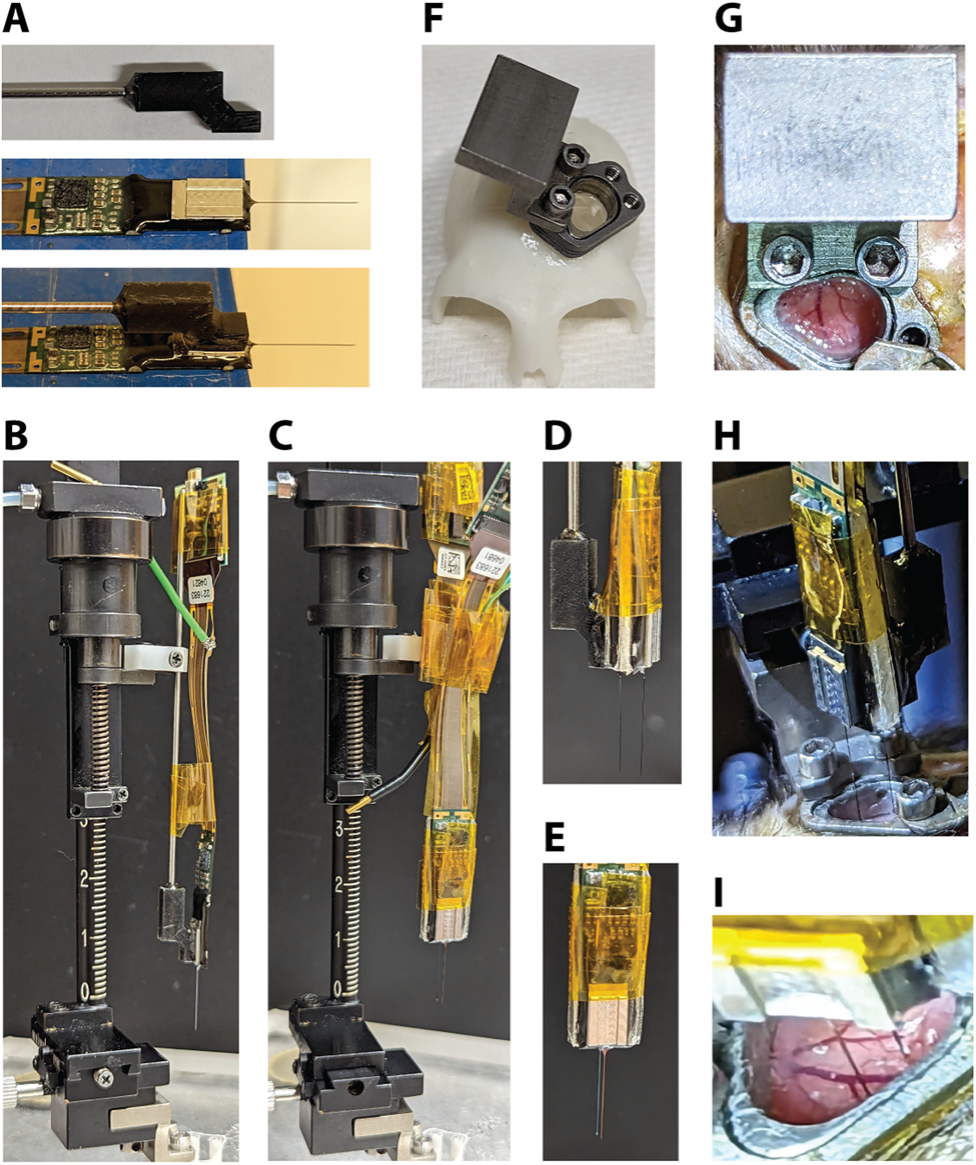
Neuropixels recording setup. *A*, A custom 3D-printed single probe holder with a metal rod is glued to the base of the Neuropixels probe. Ground and reference wires are also attached. *B*, The rod is held by the clamp of the microdrive. Tape is used to control the cable and headstage. *C*, Example of double probe setup attached to microdrive (1.0 mm spacer). *D*, Side view and (*E*) front view of double probe setup. *F*, 3D-printed skull model made from a CT scan with a chamber and insert (AD attached) and a microdrive mount attached. *G*, Photo of the microdrive mount when it is attached to the chamber. *H*, Photo of the double probe setup during the process of lowering the probes (1.9 mm spacer). *I*, Close-up view of the two probes while inserted in the cortex. Note the visible blood vessels that could be avoided during probe insertion.
